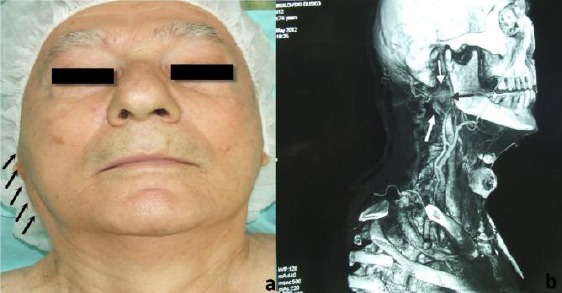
a) Oncocytoma of the right parotid gland; b) MRI of the oncocytoma in deep lobe of the parotid gland*.
